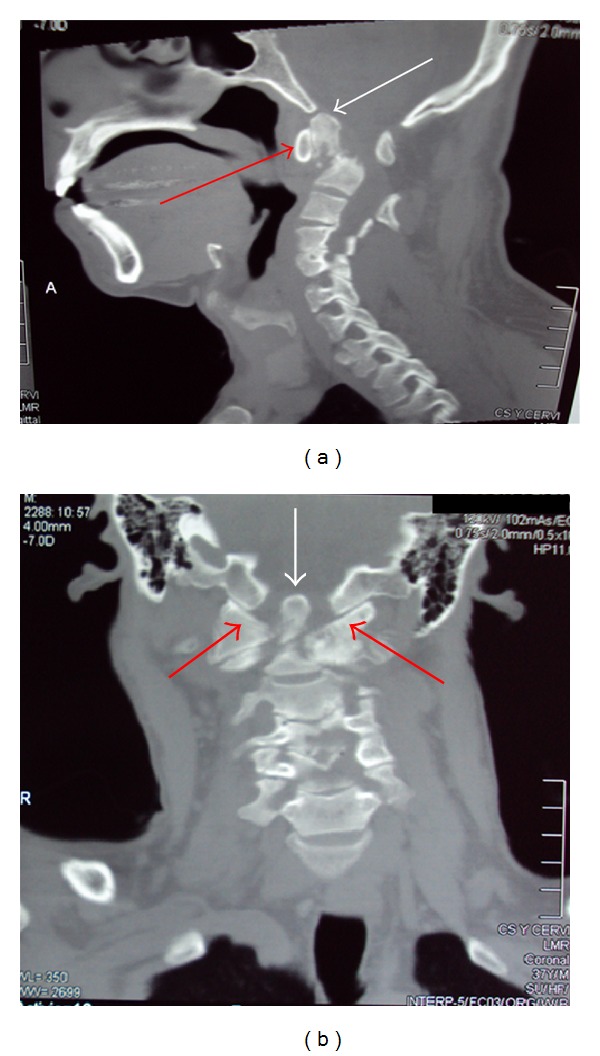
Initial CT of the cervical spine. (a) Sagittal image. Red arrow indicates the anterior arch of the C1 vertebrae (Atlas). The white arrow indicates the odontoid process fractured from the rest of the C2 vertebrae. (b) Coronal image of the initial CT. White arrow: odontoid process of C2 vertebrae with the fracture seen underneath it. Red arrow: lateral masses of the Atlas (C1 vertebrae).
